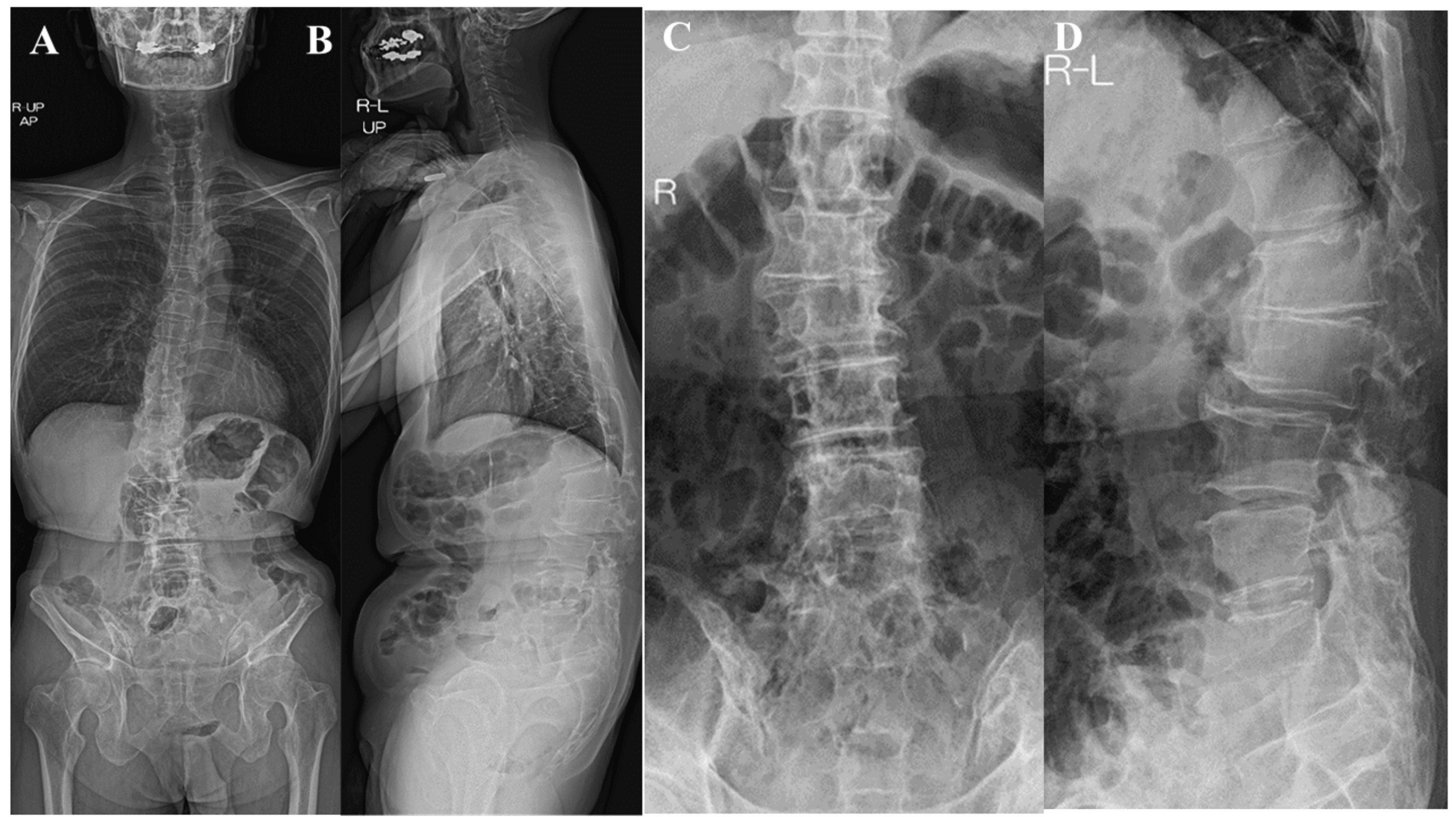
Preoperative plain X-ray imaging of the spine and hip (A) Standing anterior-posterior view of the whole spine. (B) Standing lateral view of the whole spine. (C) Preoperative plain X-ray anterior-posterior view of the lumbar spine. (D) Preoperative plain X-ray lateral view of the lumbar spine.
